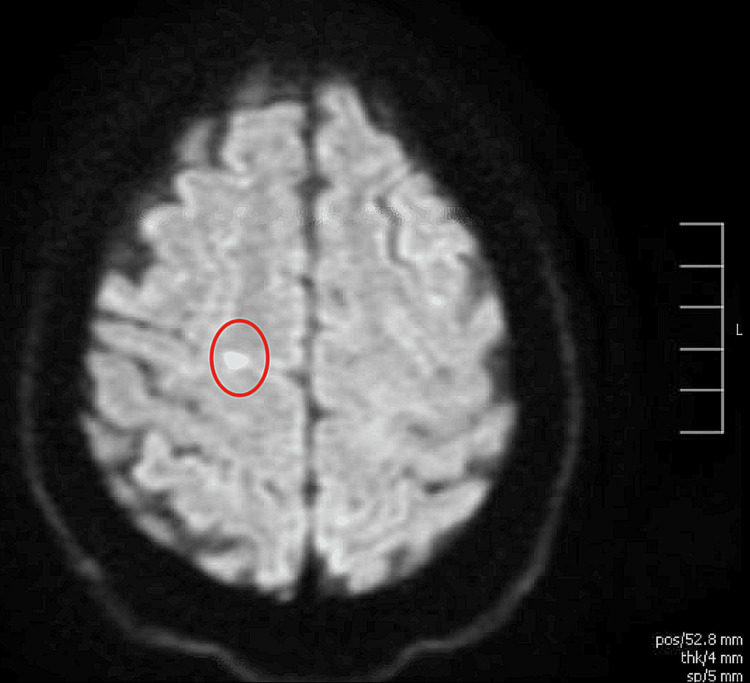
MRI of the brain showing an acute infarct in the precentral gyrus
